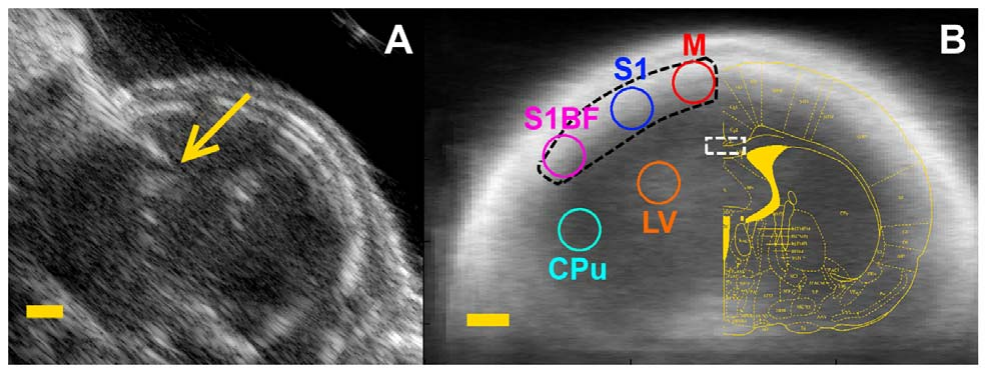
Coronal B-scan ultrasound images at the level of the LPS injection. (A) Arrow marks injection site. (B) Seeds placement, overlaid a coronal B-scan, averaged over 11 individual B-scans. Abbreviations: M, motor cortex; S1, primary somatosensory cortex; S1BF, barrel field primary somatosensory cortex; LV, Lateral ventricle; CPu, Caudate putamen. Black dotted line indicates Left Cortex ROI used for locoregional SO_2_ measurement. White dotted rectangle indicates ROI used for local SO_2_ measurement in corpus callosum. Scale bar: 1 mm.
